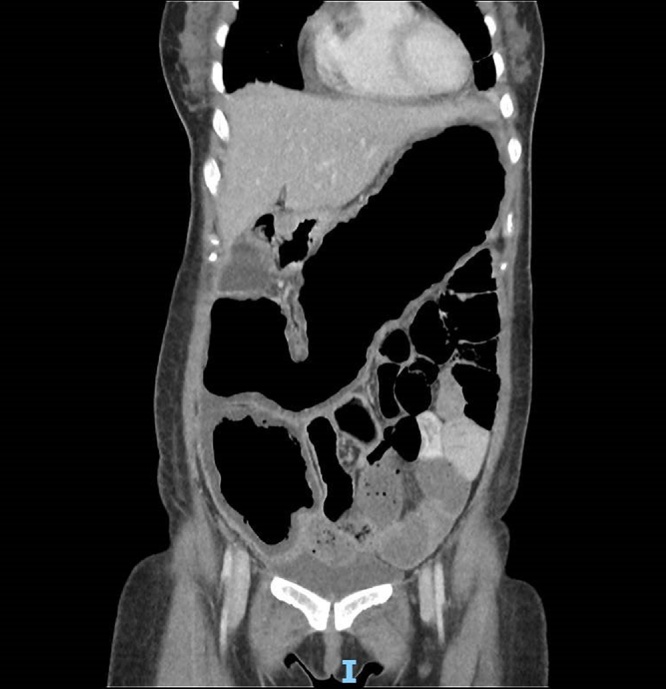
CT scan of dilated colon.
